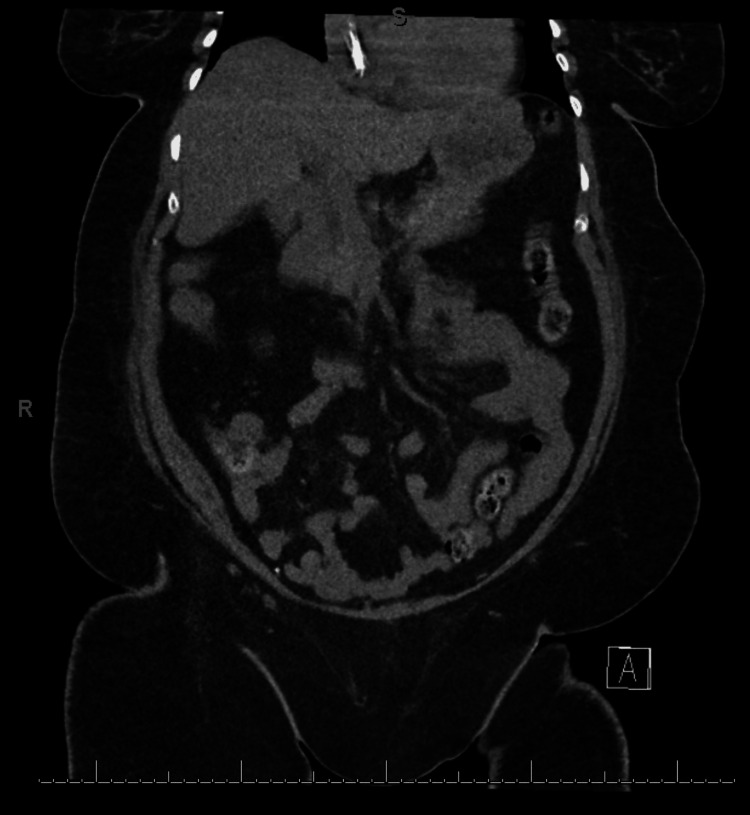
CT of the Abdomen and Pelvis Demonstrating No Acute Intra-abdominal Pathology CT - Computed Tomography
